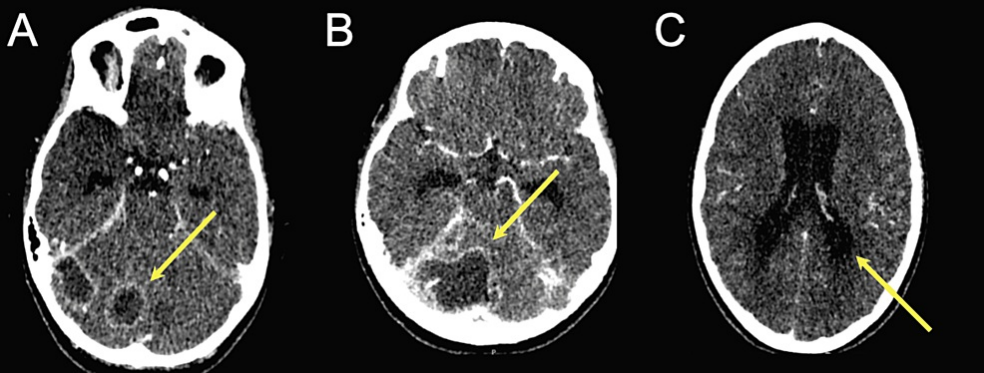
Axial CT scans revealed rim-enhanced lesions suggestive of abscess in the posterior fossa (A-B), causing hydrocephalus (C).
